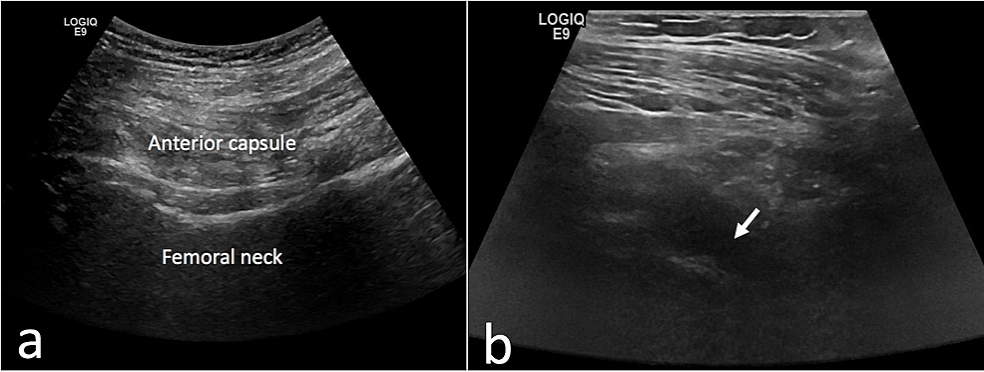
Ultrasound examination of the hip before and after the injection. Ultrasound examination of the hip with the probe at an oblique sagittal position along the femoral neck. The hyperechoic femoral head, neck, and anterior capsular recess are recognized prior to the injection with no apparent fluid collection (A). Following the intra-articular injection, there is hypoechoic fluid accumulation in the anterior femoral neck area (B).
